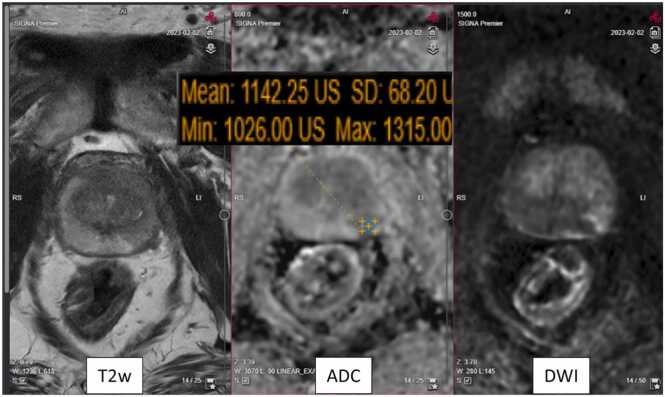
Case 10 with focal lesion in left dorsal peripheral zone. Low agreement between expert consensus (PI-RADS 3) and participants (PI-RADS 5; 19 participants and PI-RADS 4; 8 participants). Diffuse appearance of the lesion on T2 weighted transversal image (left) and only slight to moderately restricted diffusion with ADC-value 1142 × 10^−6^ mm^2^/s and DWI signal not markedly hyperintense (middle and right).
